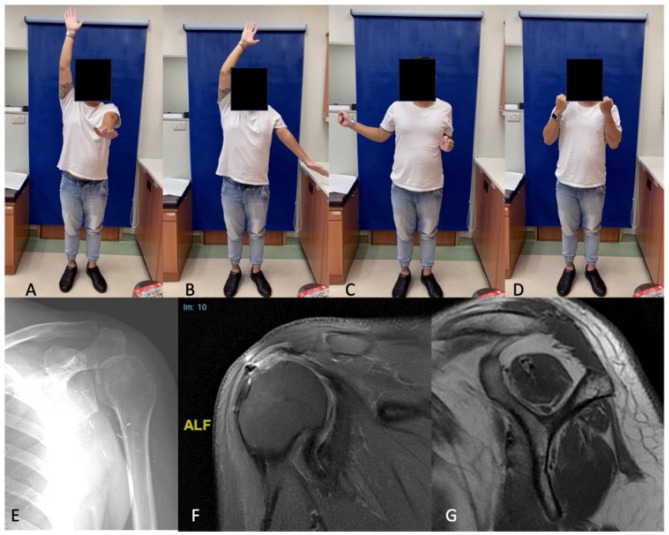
Preoperative physical and image findings of the patient. (A) The patient could only forward elevate the shoulder to 80° and (B) laterally abduct to 45°, with (C) an external rotation by the side of about 0°. (D) Elbow and wrist flexion were maintained. (E) X-ray of the left shoulder revealed a malunited proximal humerus. (F,G) MRI revealed a rotator cuff tear with Goutallier grade 1 muscle fatty infiltration.
